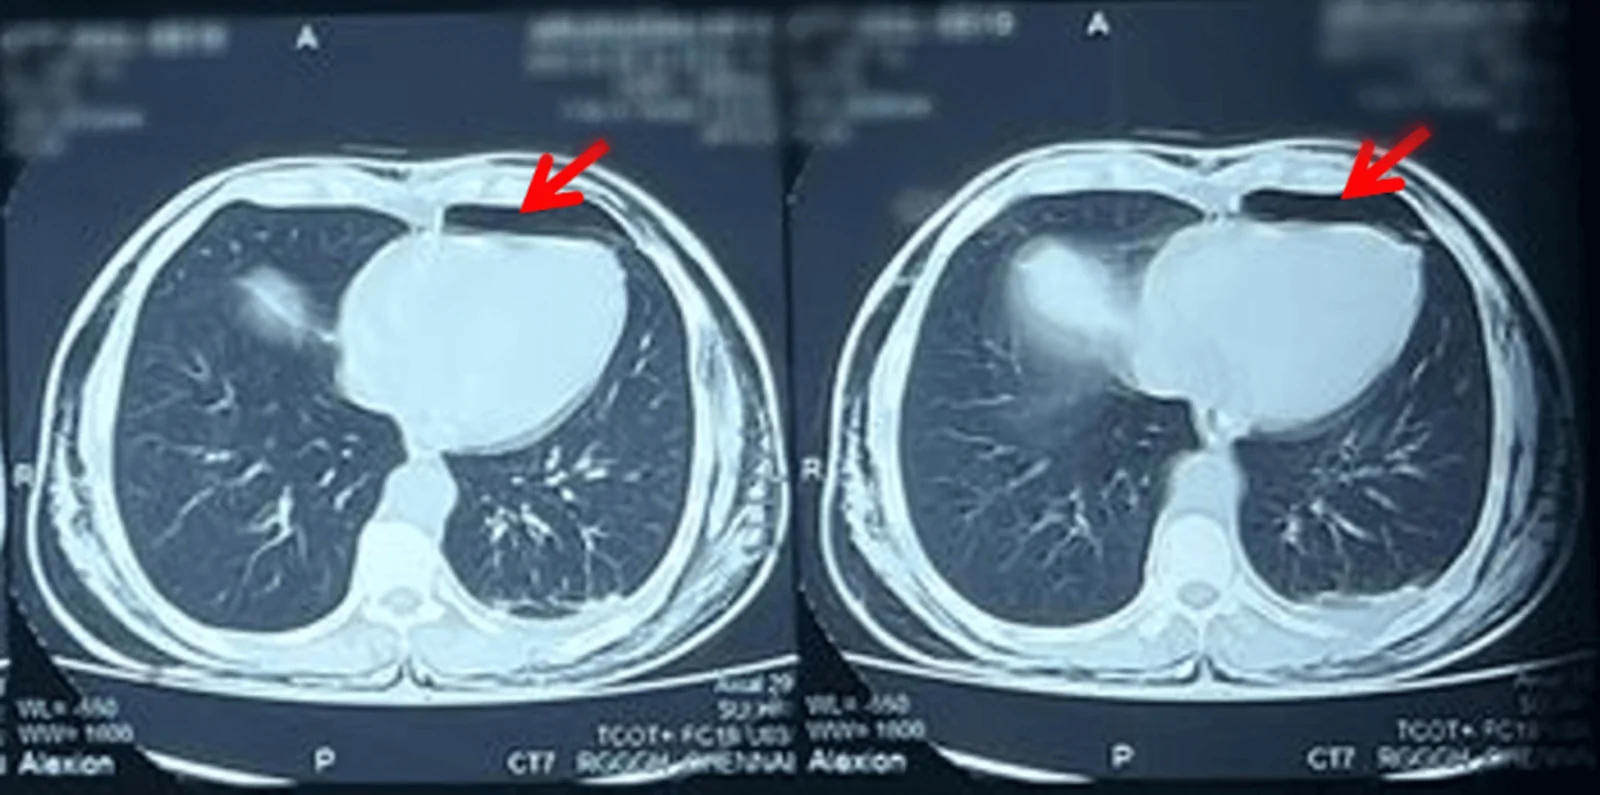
CT chest showing pneumomediastinum Axial computed tomography (CT) image of the chest, with arrows showing extensive free air within the mediastinum, confirming pneumomediastinum.
